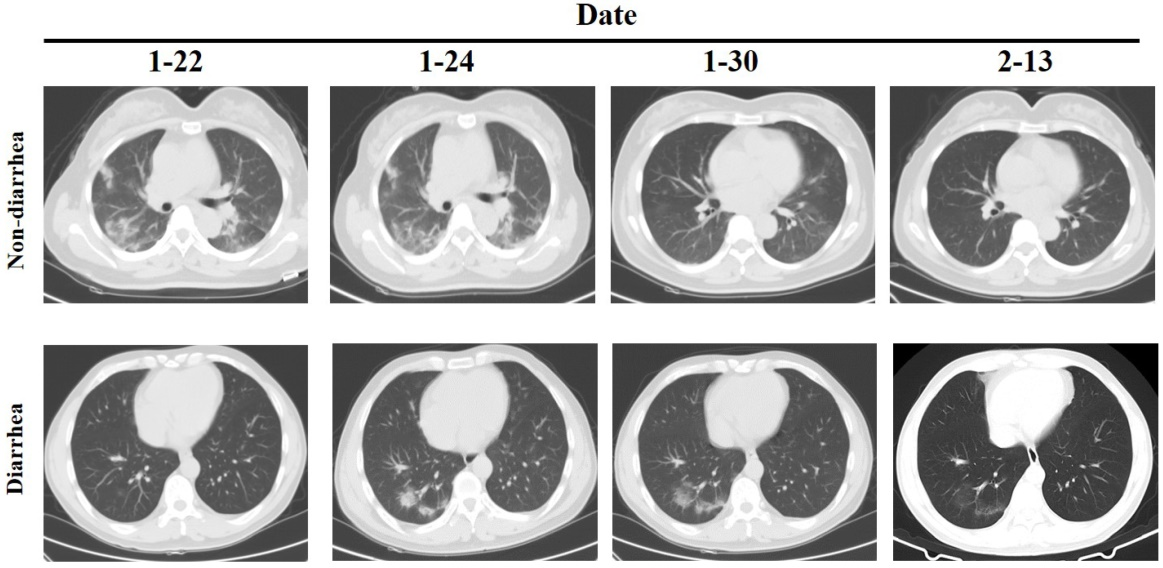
CT of chest in patients with diarrhea and non-diarrhea.
